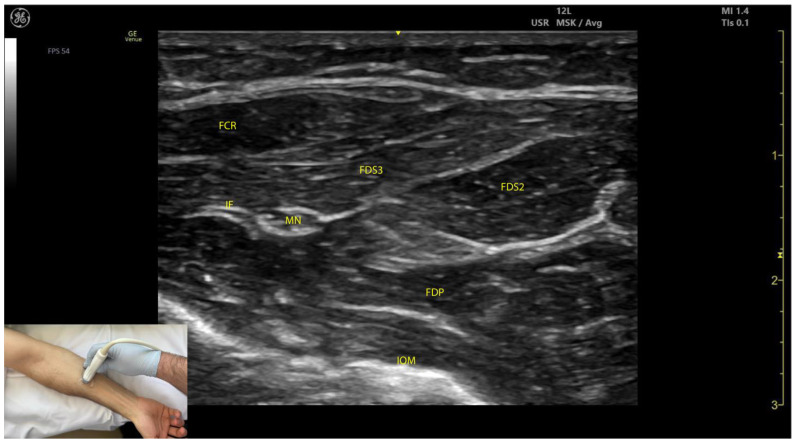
Ultrasound anatomy of the flexor digitorum superficialis (FDS) with key landmarks: FCR—flexor carpi radialis; FDS3—flexor digitorum superficialis digit 3; FDS2—flexor digitorum superficialis digit 2; IF—intermuscular fascia; FDP—flexor digitorum profundus; IOM—interosseous membrane; MN—median nerve.
